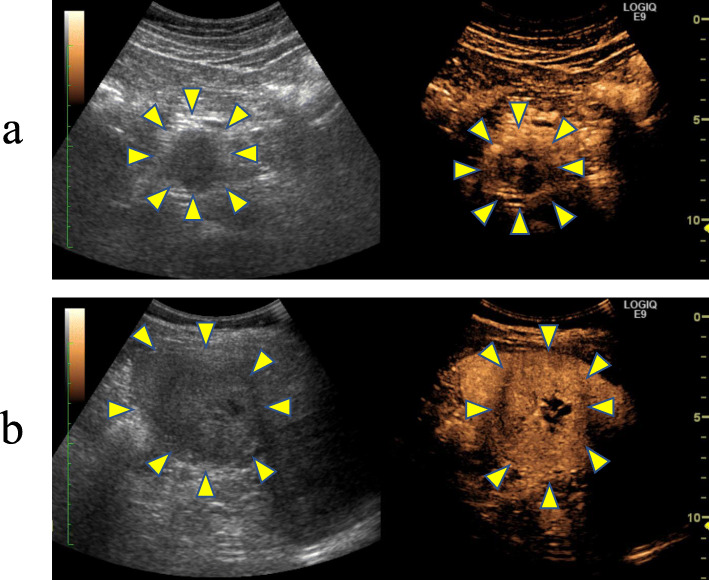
Abdominal ultrasonography (US) with and without contrast. Abdominal US with and without contrast revealed the presence of isodense masses of 34 mm (a) in the pancreatic head and 60 mm and in the spleen (b) (arrows)
